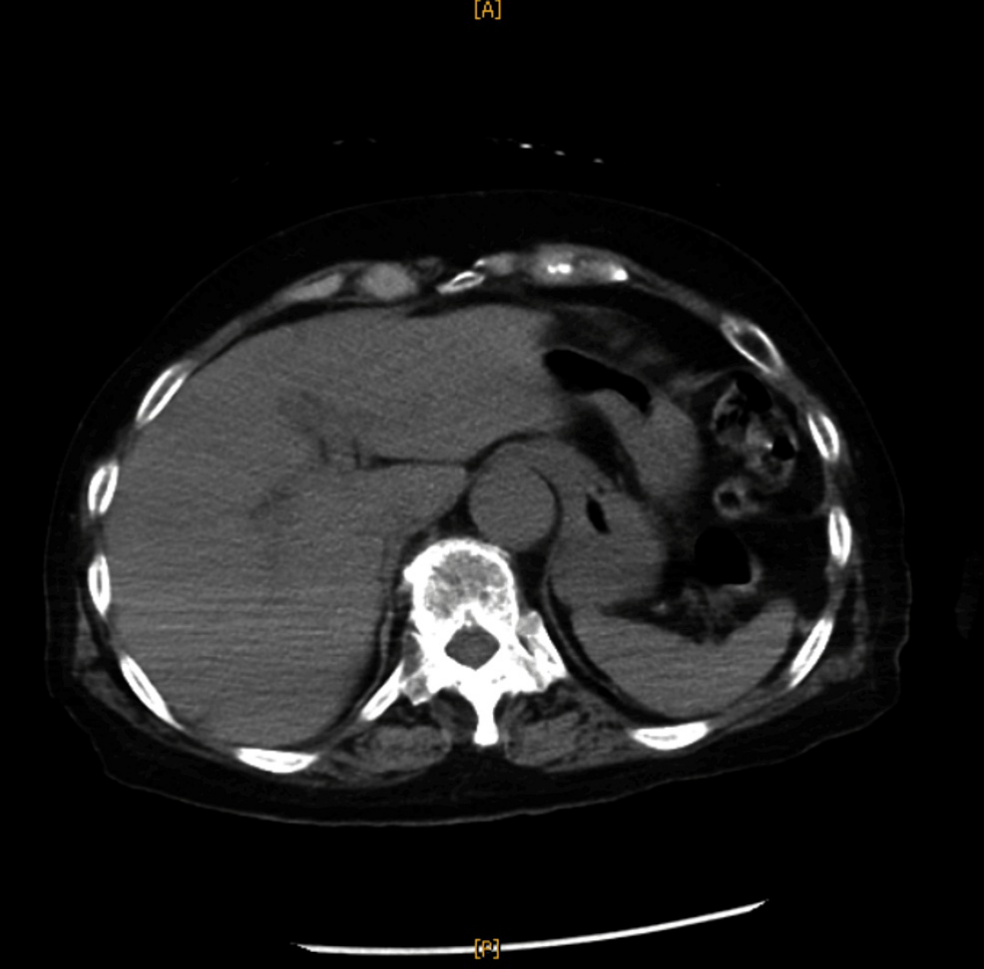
Plain CT of the upper abdomen There was no abnormality in the patient's liver.
